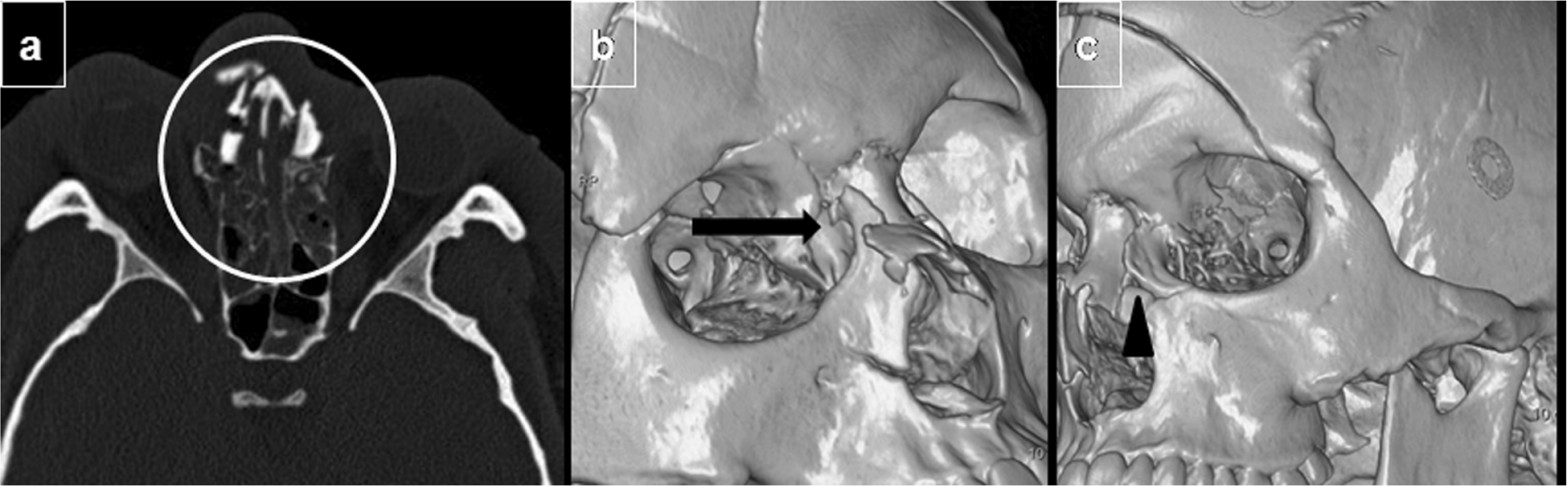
Bilateral naso-orbito-ethmoidal fracture. a Axial unenhanced CT image at the level of the mid-orbit shows a comminuted fracture of the naso-orbito-ethmoid complex (circled) resulting in telecanthus (widened intercanthal distance). b, c Oblique 3D reconstructions to assess the insertion of the medial canthal tendon on the bones of the lacrimal fossa: more significant comminution with an avulsed fragment can be seen on the right (arrow) than on the left side (arrowhead)
